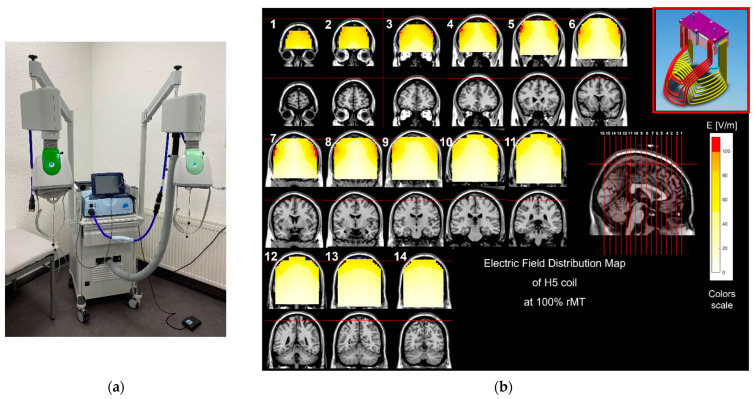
(a) Deep TMS system at the Department of Neurology in Kempen with Brainsway H5 Coil CE-marked for Parkinson’s (light green, right) and H2 Coil CE-marked for Alzheimer’s (dark green, left); Magstim Stimulator and Air-cooling. (b). Distribution of electric field induced by the H5 coil. The electric field distribution was measured in a model of the human head (15 × 13 × 18 cm) filled with physiologic saline solution. The colored field maps indicate the electric field absolute magnitude in each pixel, for 14 coronal slices, 1 cm apart, along with the appropriate MRI coronal images. The H5 coil was placed over the theoretical frontal cortex of the head model and the field in each pixel was measured using a ‘pick-up’ dipole probe attached to an oscilloscope. The red pixels indicate field magnitude above the threshold for neuronal activation, which was set to 100 V/m based on the average threshold for motor activation of the hand. The field maps are adjusted to obtain 100% of the threshold at a depth of 1.5 cm (Image provided by Brainsway).
